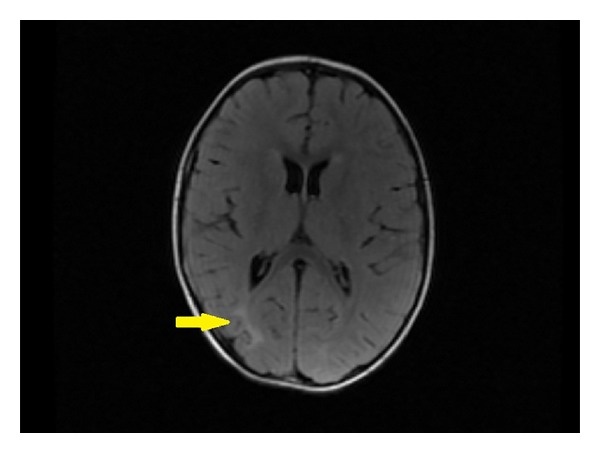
Brain MRI showed a subcortical increased signal in the right occipital lobe on T2-weighted image.
